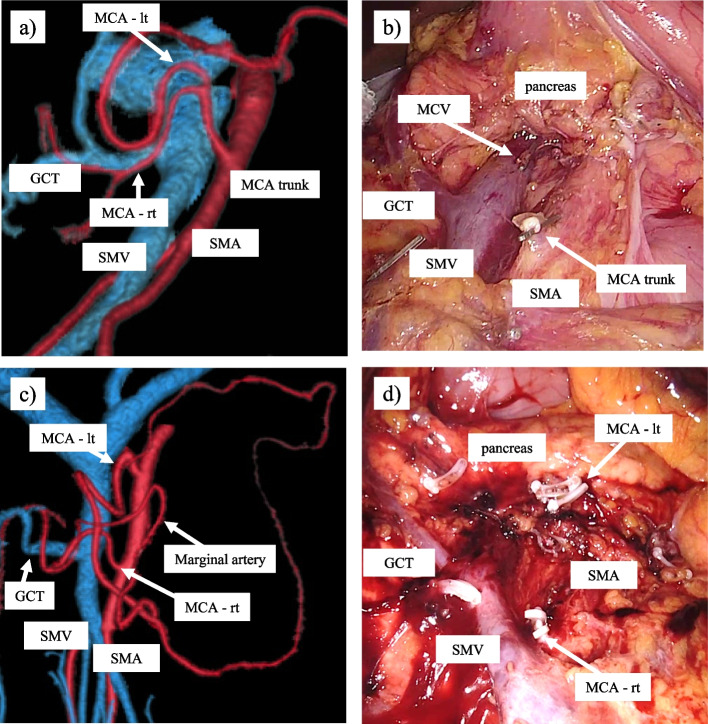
CT angiography and intraoperative findings of type I and type IIa MCA bifurcation. a CT imaging of a type I MCA bifurcation. The right and left branches of the MCA branch from the common trunk. b Intraoperative findings after main lymph node dissection by cutting the common trunk for extended right hemicolectomy in a case with a type I MCA. c CT imaging of a type IIa MCA bifurcation. The right and left branches of the MCA branch separately from the SMA. d Intraoperative view after main lymph node dissection with ligation of the right and left branches of a type IIa MCA for extended right hemicolectomy. CT, computed tomography; MCA, middle colic artery; MCV, middle colic vein; SMV, superior mesenteric vein; GCT, gastrocolic trunk
